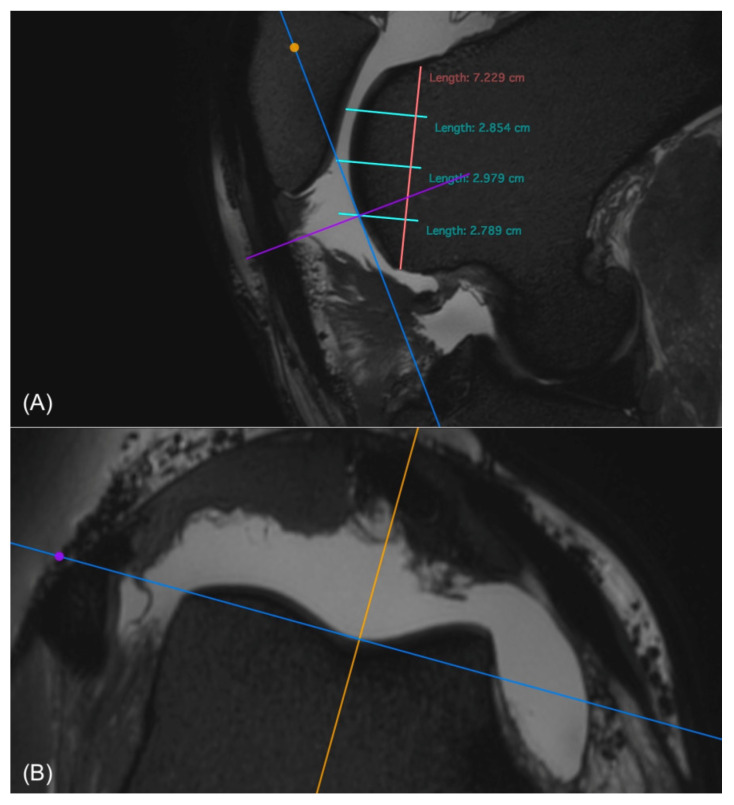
(A) Sagittal and (B) transverse view of the trochlear groove in a 3D T2-weighted image where the regions of interest (ROIs) were determined. The ROIs are marked as described in Figure 1 and Figure 2 (A). To measure the cartilage thickness in the transverse image (B) at the same ROI, the cross of the transverse (purple) and dorsal (blue) planes was placed at the ROI in the sagittal view (A). This automatically places the plane cross on the same ROI in the transverse view (B). Care was taken to place the transverse plane (purple) perpendicularly to the cartilage surface at the point of measurement. This was carried out for all ROIs in the femoropatellar joint. For the femorotibial joint the dorsal view was used instead of the transverse.
